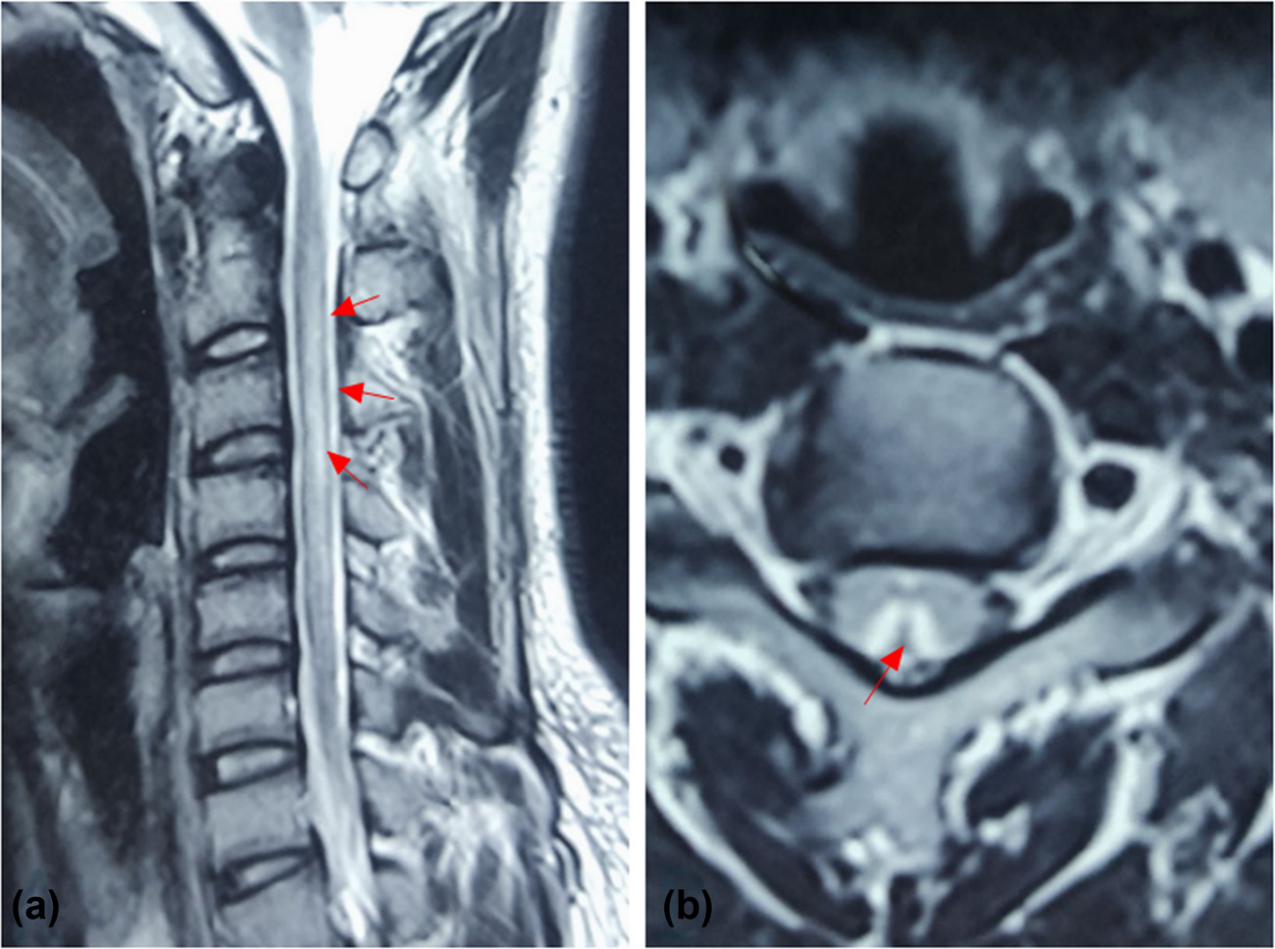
Cervical MRI T2WI images of a 19-year-old male patient showing abnormal signals of the cervical spinal cord in sagittal view (indicated by red arrow, a), and typical “inverted V sign” of the cervical spinal cord in transverse view (indicated by red arrow, b).
